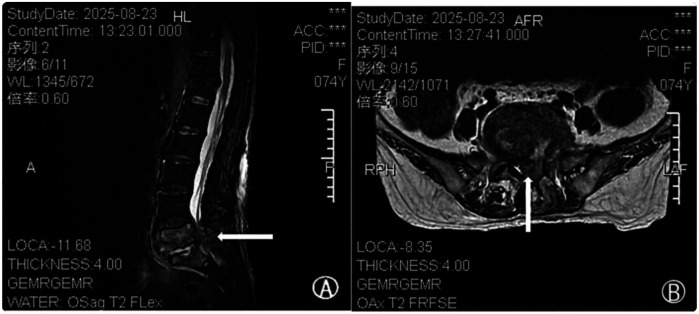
Lumbar MRI showing reduction of the intraspinal abscess.
